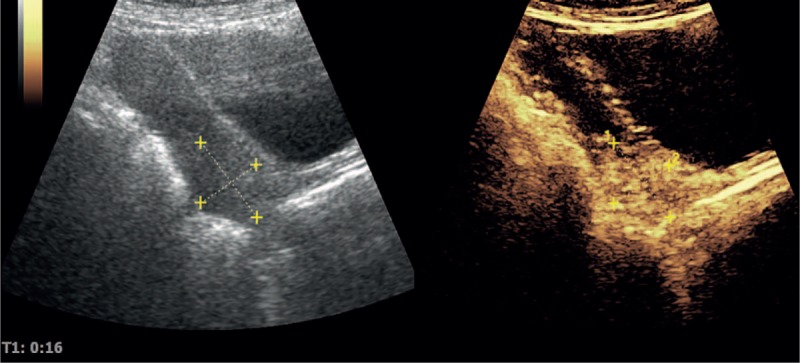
The enhanced pattern by contrast-enhanced ultrasound (CEUS) displayed strong peripheral enhancement accompanied with globular appearance and then centripetal filling completely.
